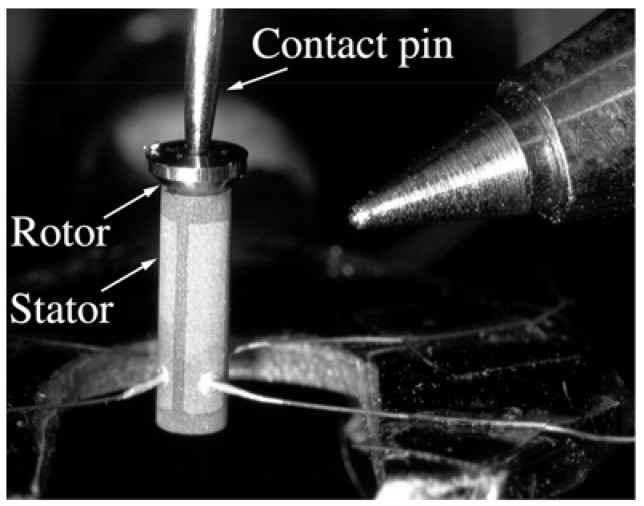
Cylindrical-shaped micro ultrasonic motor using polycrystalline PZT film [17].
